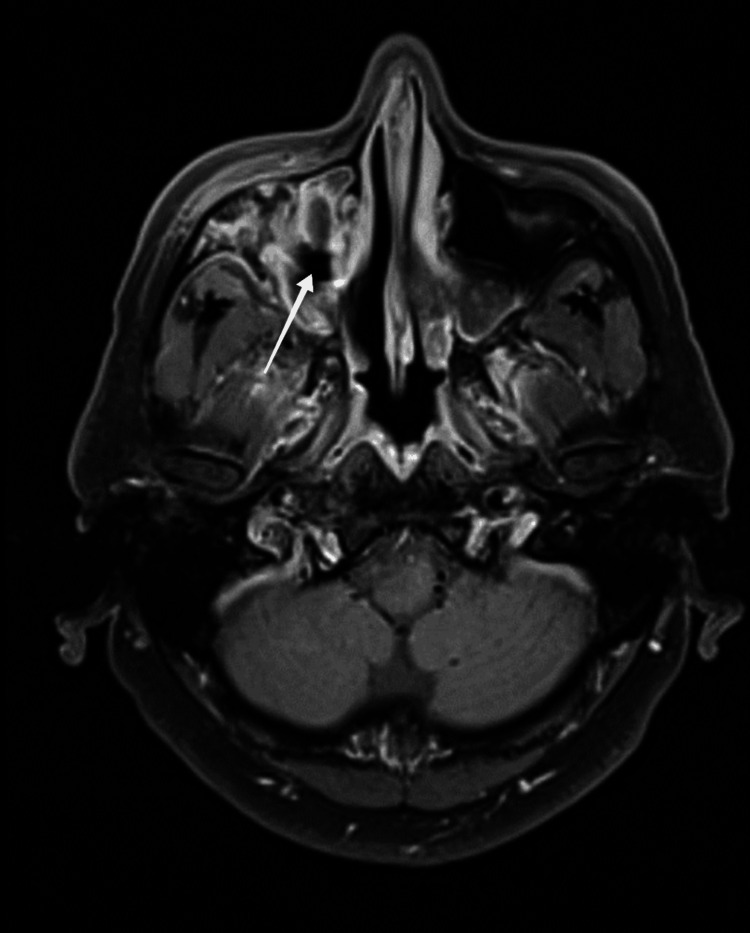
Axial T1-weighted post-contrast image showing a heterogeneous enhancement pattern in the right maxillary sinus and premaxillary region.
